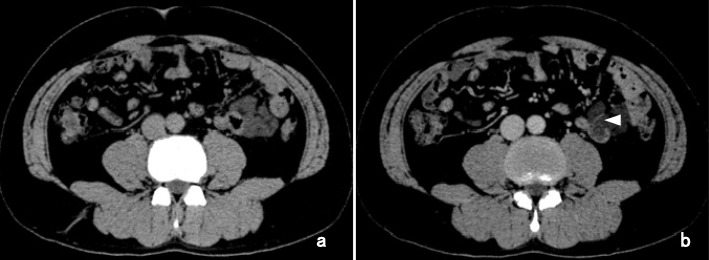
Findings of the CT scan performed in February. a A low-density mass showing an irregular outline and measuring 45 × 42 mm in size was detected; the mass was in contact with the jejunum in the left abdomen at the slightly cranial level of the umbilicus, as seen on plain CT. The oral side of the jejunum did not show dilatation. b In the contrast-enhanced image, the tumor lacked contrast. The tumor involved the peripheral artery (white arrowhead), indicating that the tumor developed in the mesentery of the jejunum; however, the adjacent jejunum showed good enhancement
